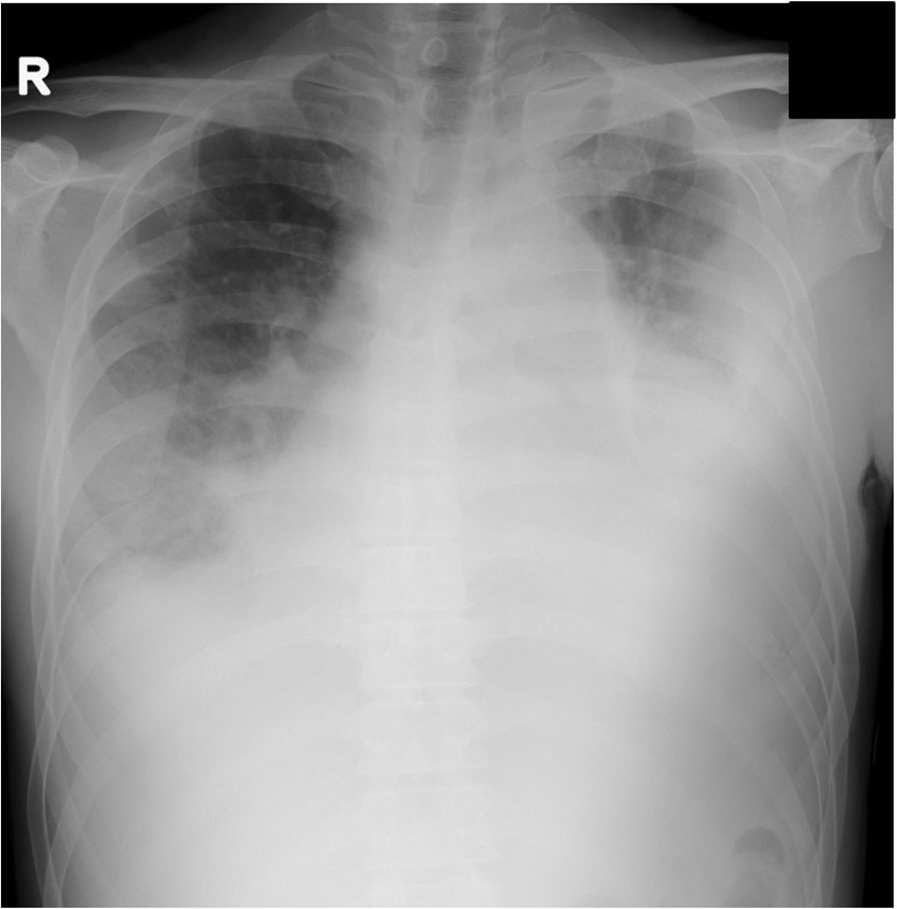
The chest radiograph revealed a mass in the left upper lung, bilateral pleural effusions and cardiac enlargement
